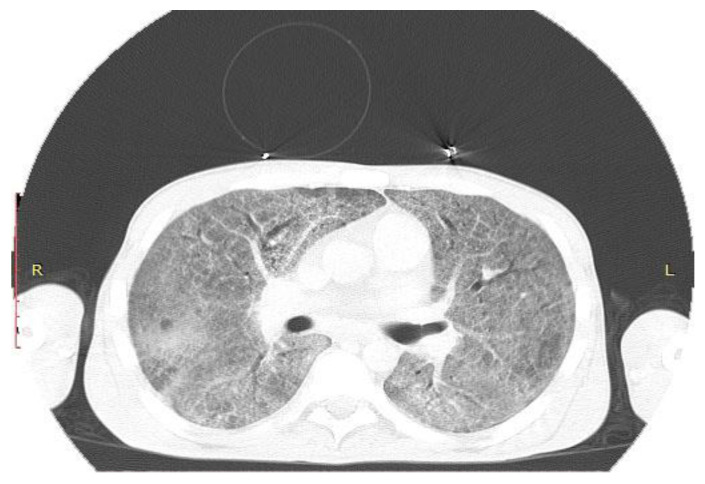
Aspects of pulmonary CT-ground-glass opacities. Repeated pulmonary CT scan (after three weeks) showing the same aspects.
